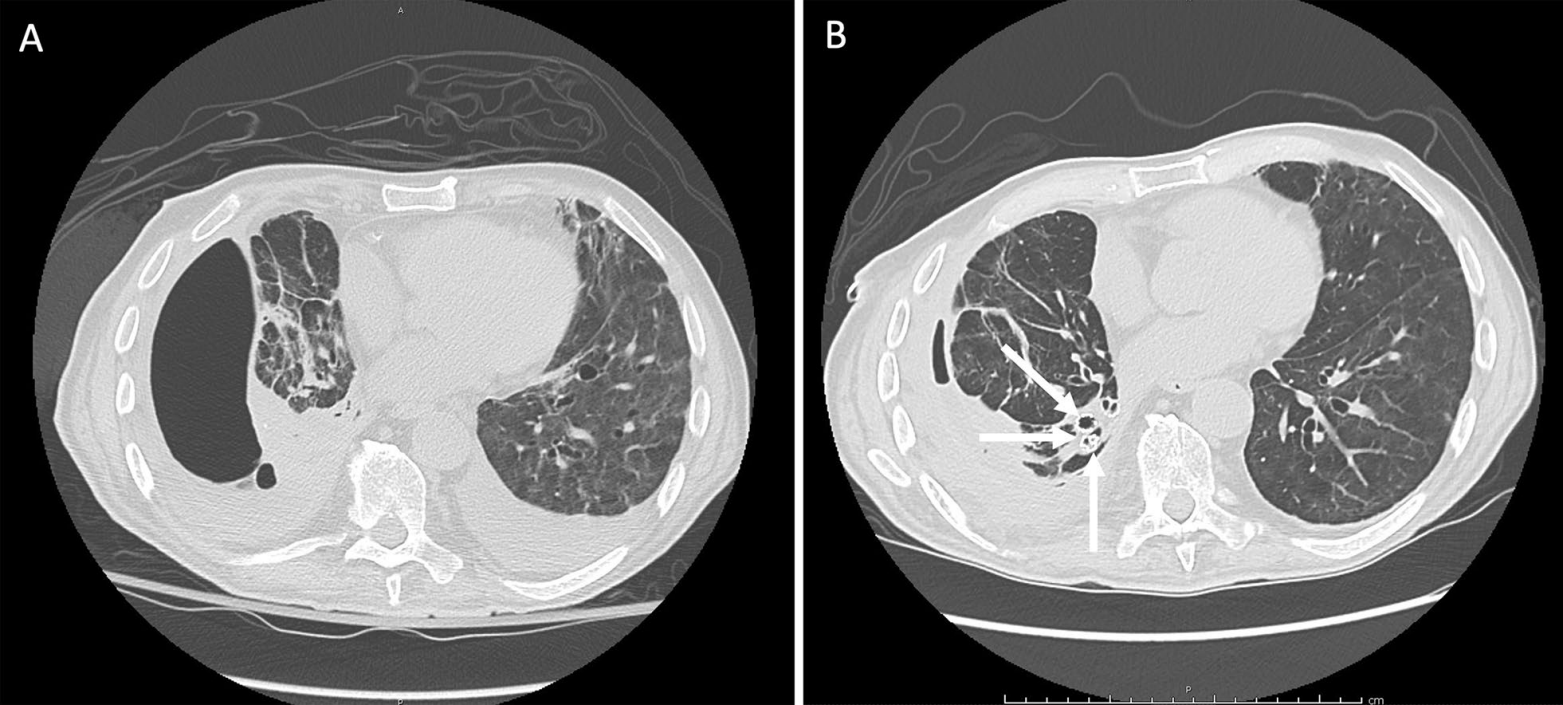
High resolution computed tomography scan in Case 2 showing right pneumothorax secondary to alveolar-pleural fistula (APF) before (panel A) and after (panel B) endobronchial valve (EBV) positioning with lung parenchyma re-expansion. Valves can be observed in the basal pyramid bronchi (withe arrows)
